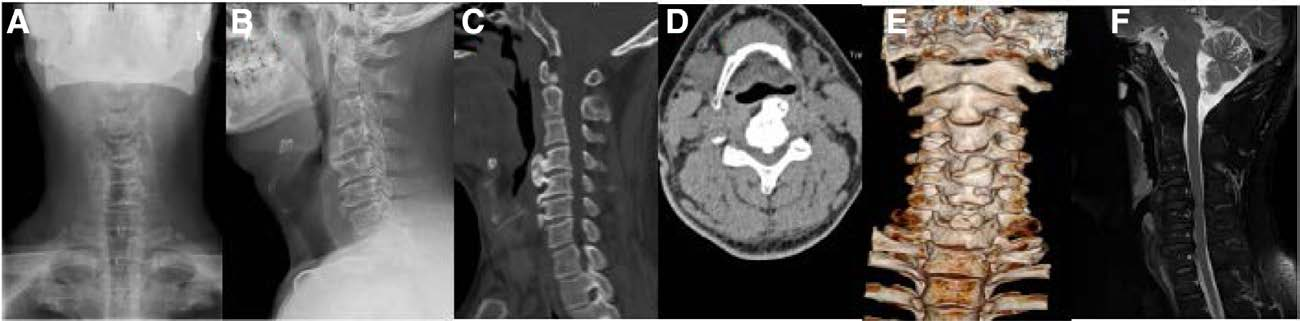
(AB) Anterior and lateral positions of cervical spine show huge osteophytes in front of C4 and 5 segments; (CD) CT of cervical spine showed anterior airway stenosis; (E) Three dimensional reconstruction of cervical spine; (F) C4/5 intervertebral disc protrusion can be seen on MRI of cervical spine.
